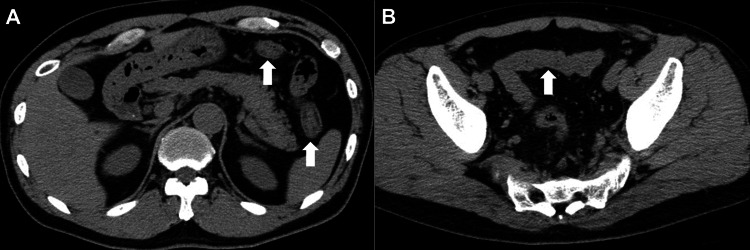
Computed tomography images acquired from Case 1. Thickening of the wall from the transverse colon (A, arrows) to the rectum (B, arrow).
